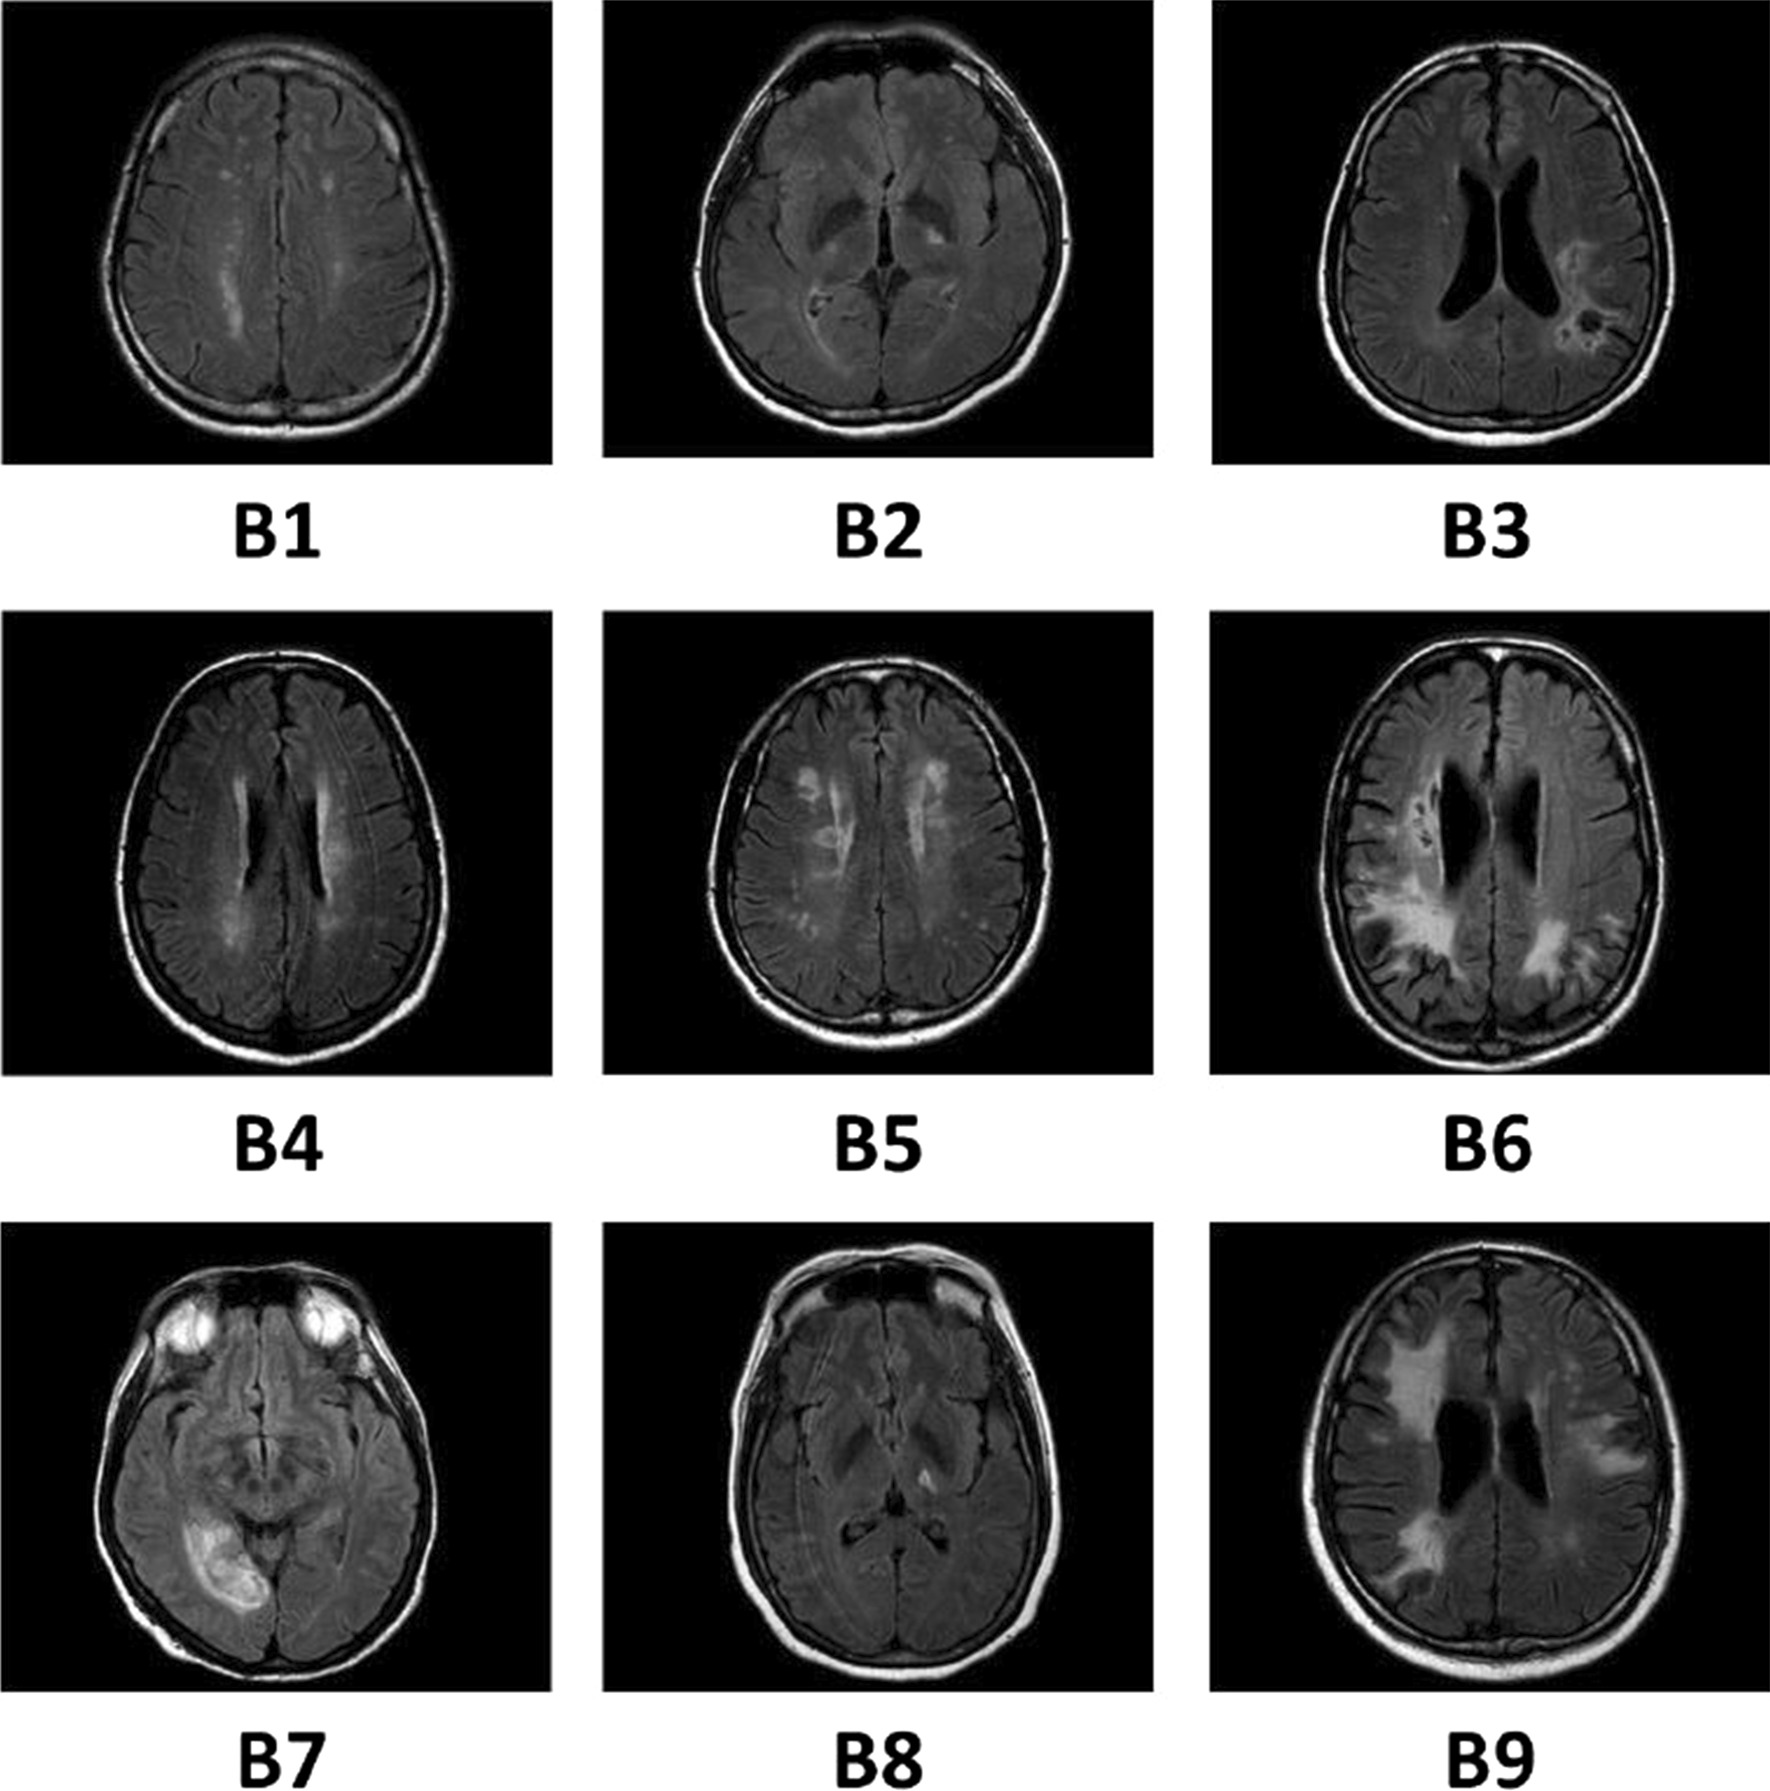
Individual FLAIR images among stroke patients in IntelliRehab in the axial view. The panel B1 to B9 shows the slice with maximum infarct volume/hyperintensities. Each subject was coded by a serial number
